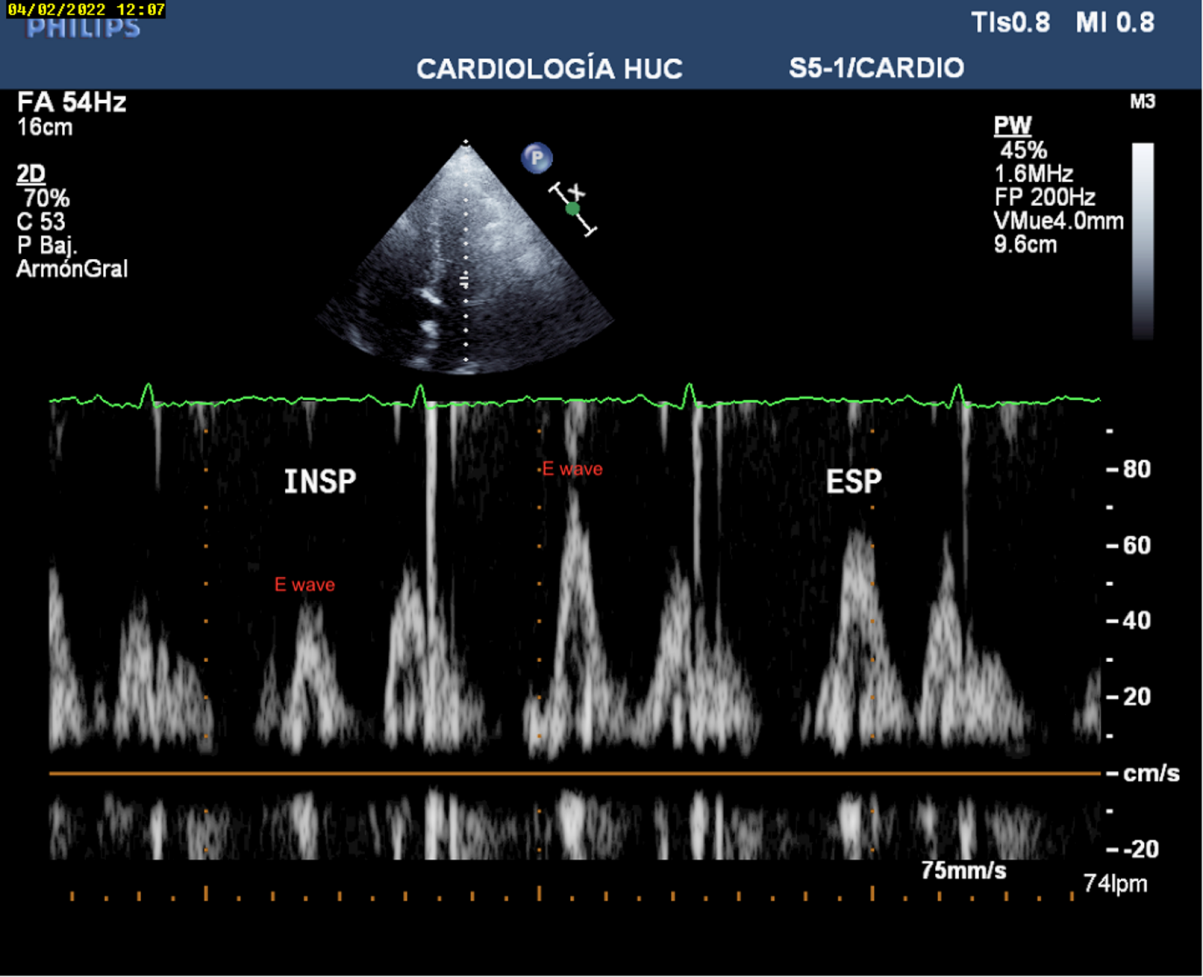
Transmitral doppler assessment. An E‐wave velocity decrease over 25% with inspiration can be observed.
